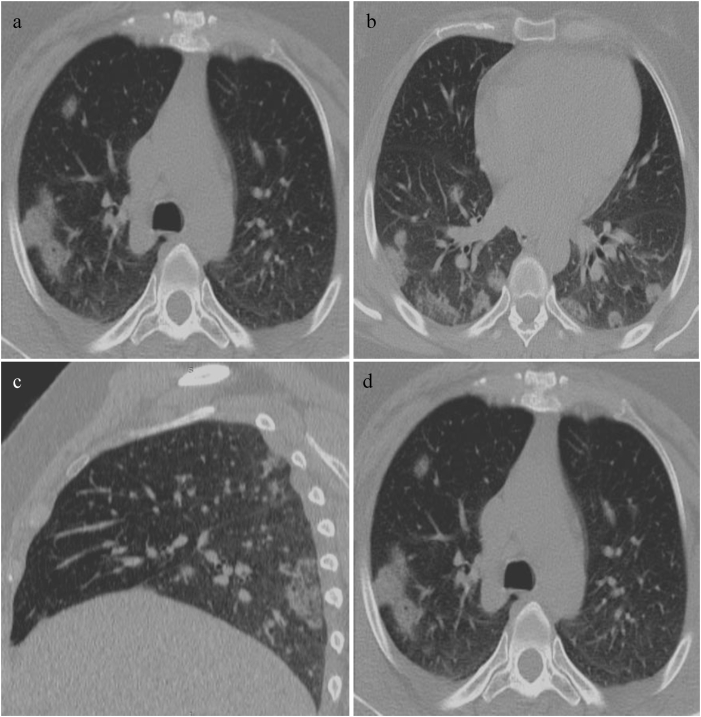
Typical Covid-19 imaging features in a 45-year-old woman with a positive RT-PCR. Unenhanced axial (a, b), sagittal (c) and coronal (d) CT images of the lung show multifocal, bilateral, posterior and peripheral rounded consolidations surrounded by ground glass opacities.
